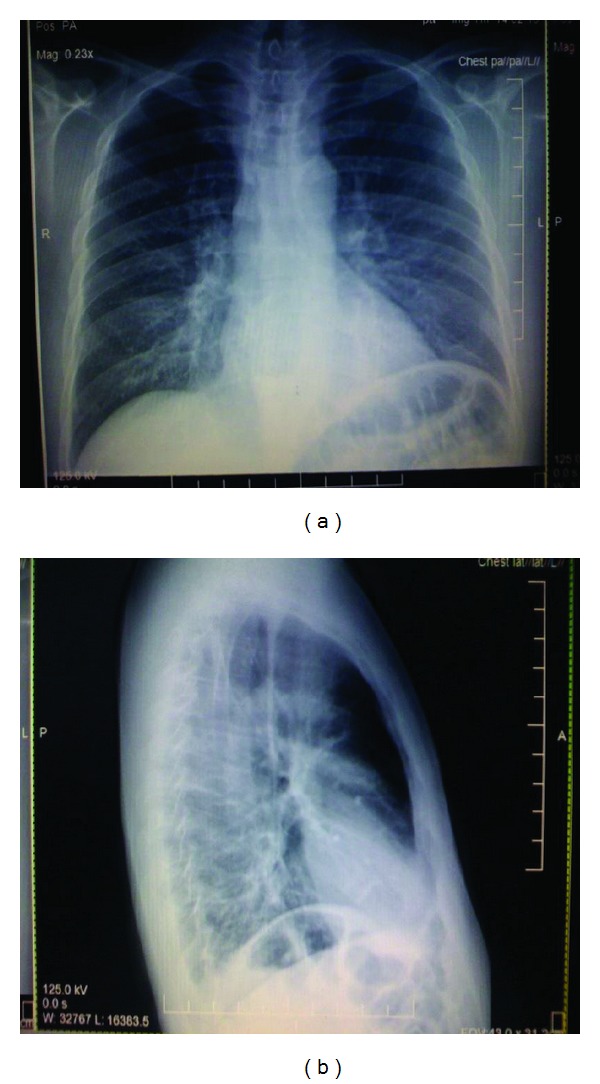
Posterioranterior and lateral chest radiographs showing dilation of the esophagus.
